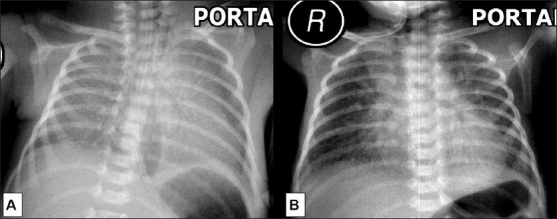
Wet lung (transient tachypnea of the new born): Frontal chest radiograph (A) on day 1 at 6 hours shows a bilateral diffuse ground-glass appearance and fine granularity due to interstitial opacities. Note the left paraspinal lucency which is due to air within the distal esophagus. Frontal chest radiograph (B) of the same patient at the age of 2 days (B) shows that the pulmonary parenchymal opacities have decreased, though perihilar streaky markings are still present
